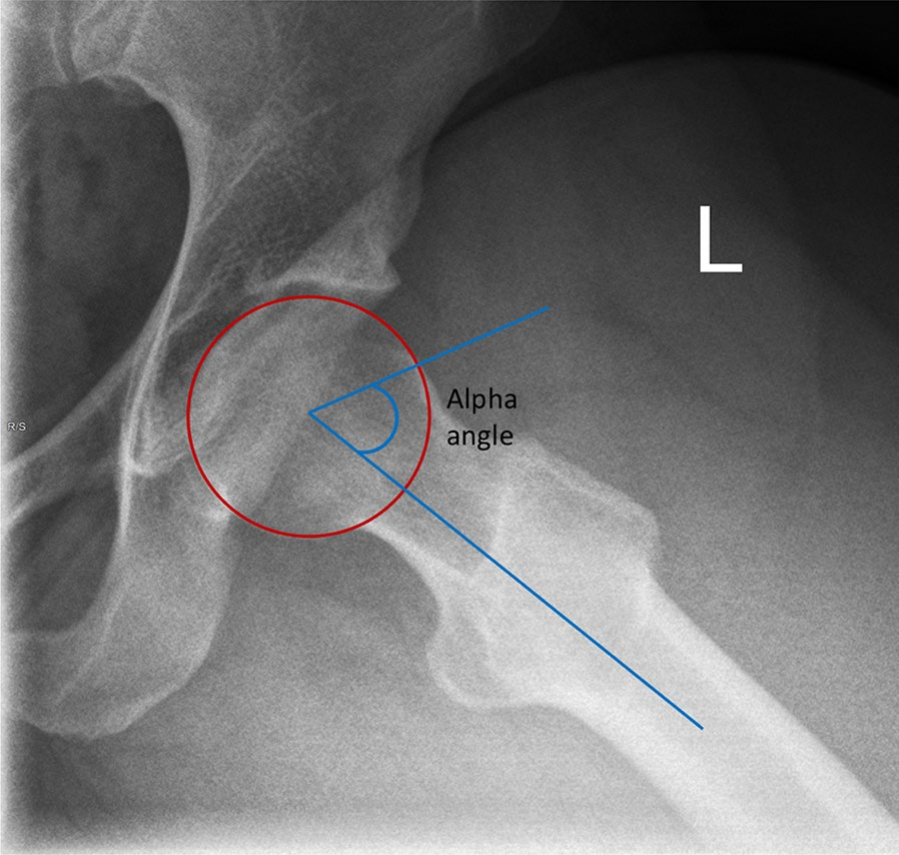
Alpha angle on lateral plain radiograph of left hip
